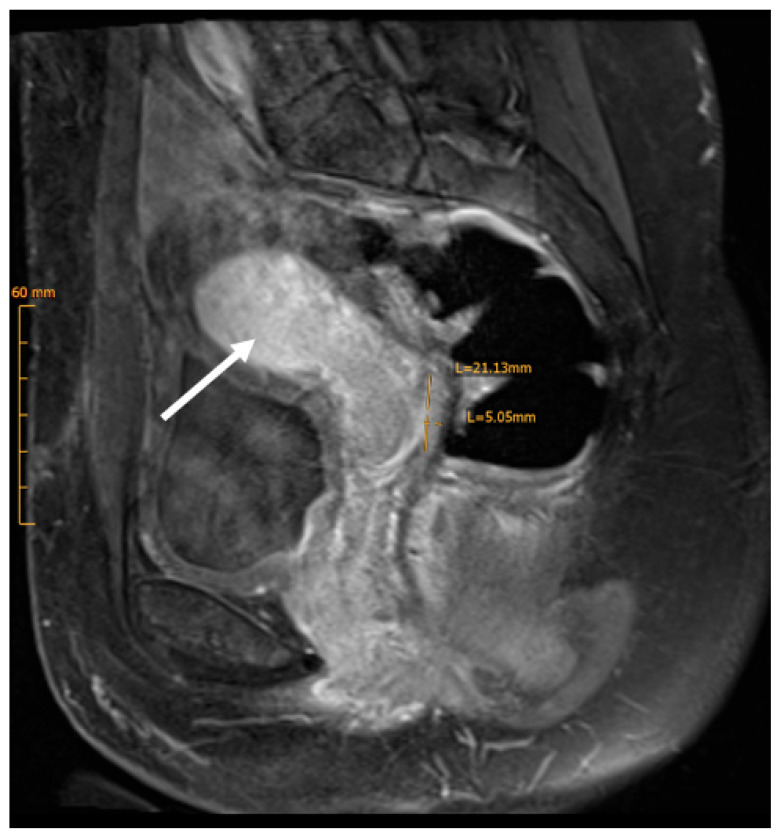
Postoperative MRI demonstrating post-radiation uterine changes. MRI shows a preserved uterine contour with diffusely low T2 signal in the myometrium and partial blurring of the junctional zone, compatible with post-radiation change, as well as a thin endometrium (white arrow).
